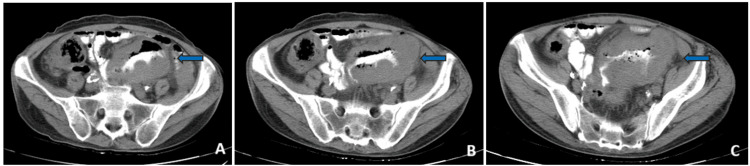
Thoracoabdominopelvic CT scan, transverse cuts A, B, and C: superior to inferior transverse cuts; Blue arrows: abdominal mass with an ileocolic fistula
